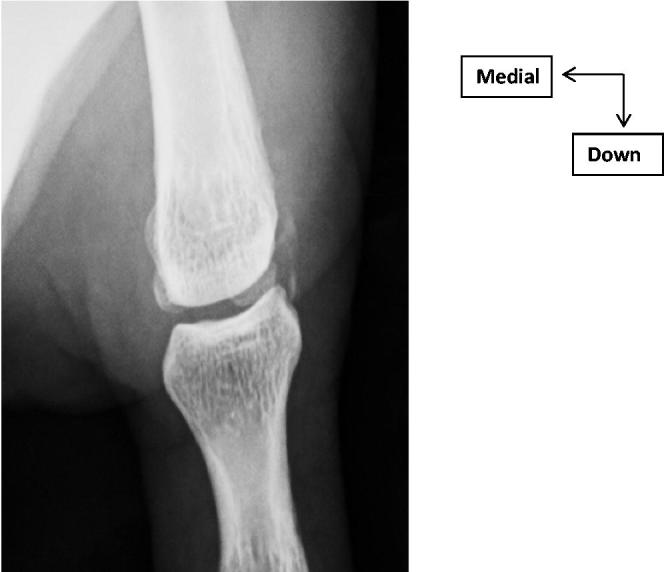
Inches x-ray: calcification of LCL of MCPP.
